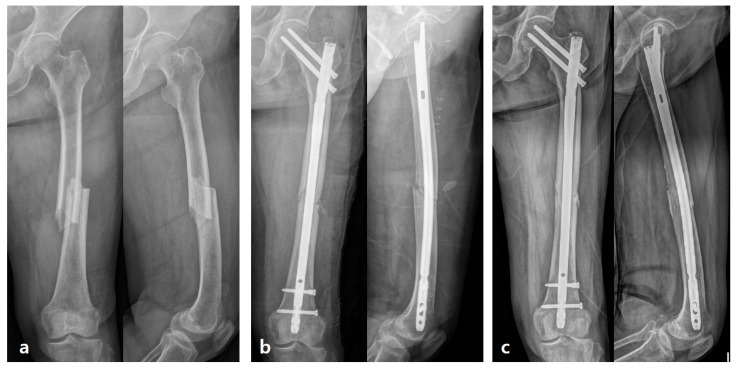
Serial anteroposterior and lateral radiographs of a 77-year-old woman. (a) Preoperative radiographs show a diaphyseal complete atypical femoral fracture, (b) Immediate postoperative radiographs after fixation with an IM nail and decortication at the fracture site, (c) Radiographs at 4 months postoperatively demonstrate complete cortical bridging and fracture union.
